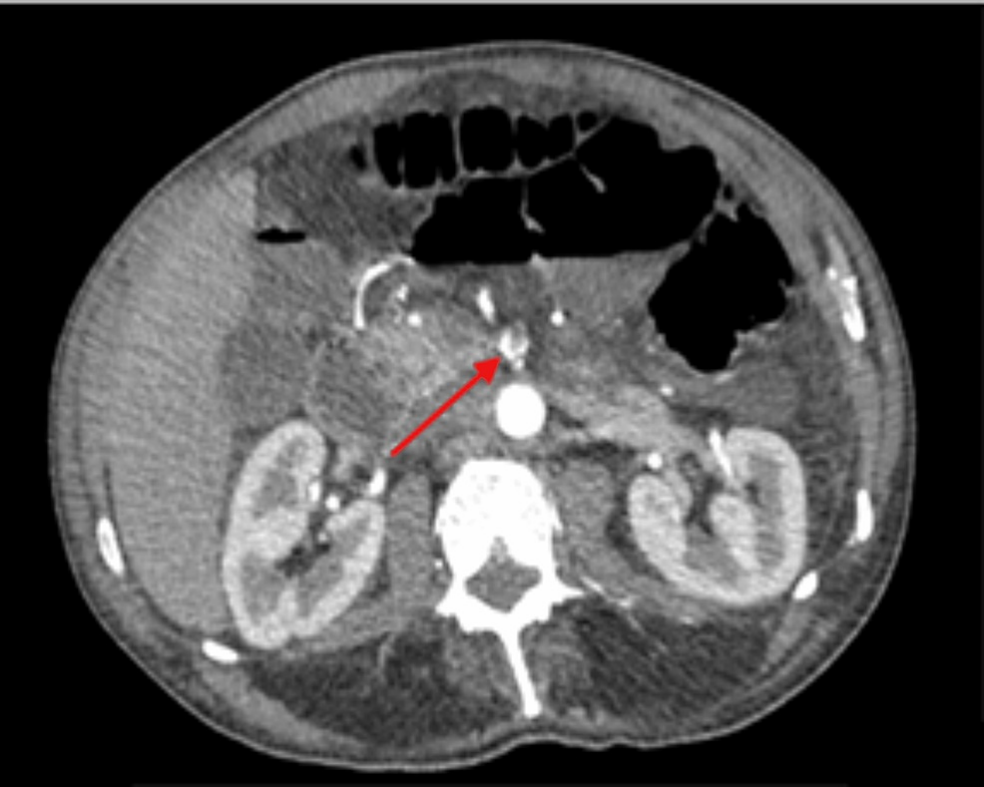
Transverse plane contrast CT of the abdomen and pelvis, with a red arrow depicting partial thrombosis of the superior mesenteric artery. Distended, fluid-filled bowel loops are also visualized.
